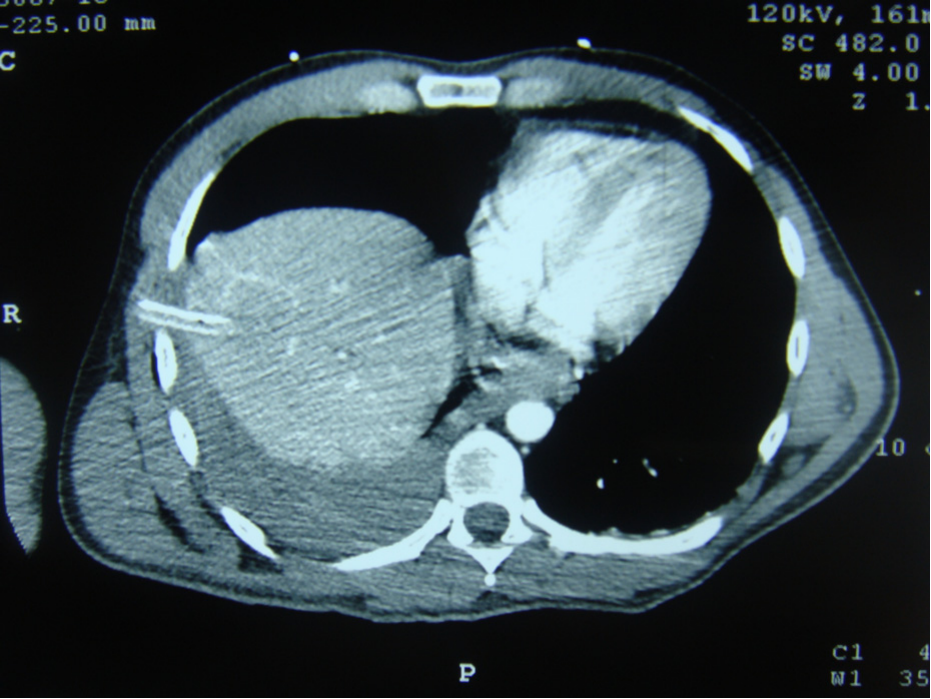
CT scan of the patient where hepatothorax is displayed with the drain inside.
